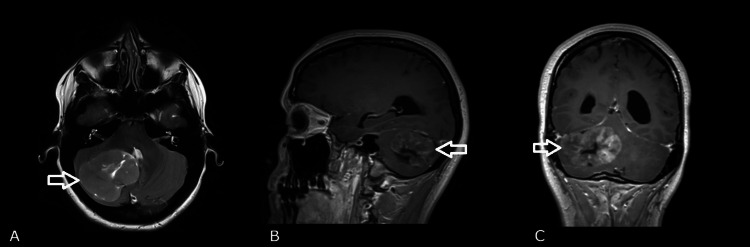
Preoperative magnetic resonance imaging showing a lesion (white arrow) in the right cerebellar hemisphere with axial dimensions of 51 x 48 mm (A: axial; B: sagittal; C: coronal).
